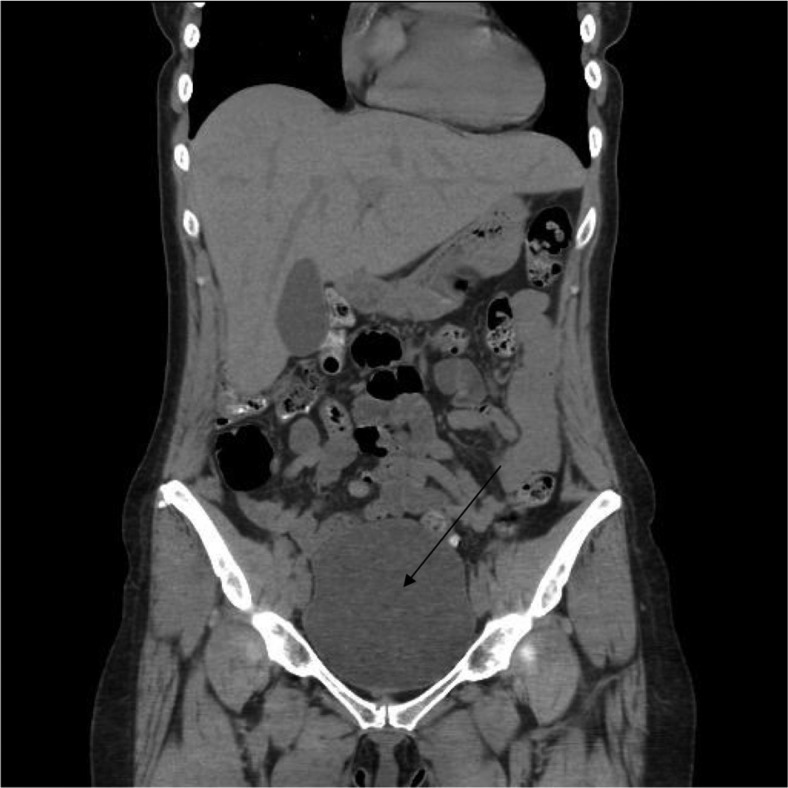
CT scan demonstrating distended urinary bladder in the pelvis.
